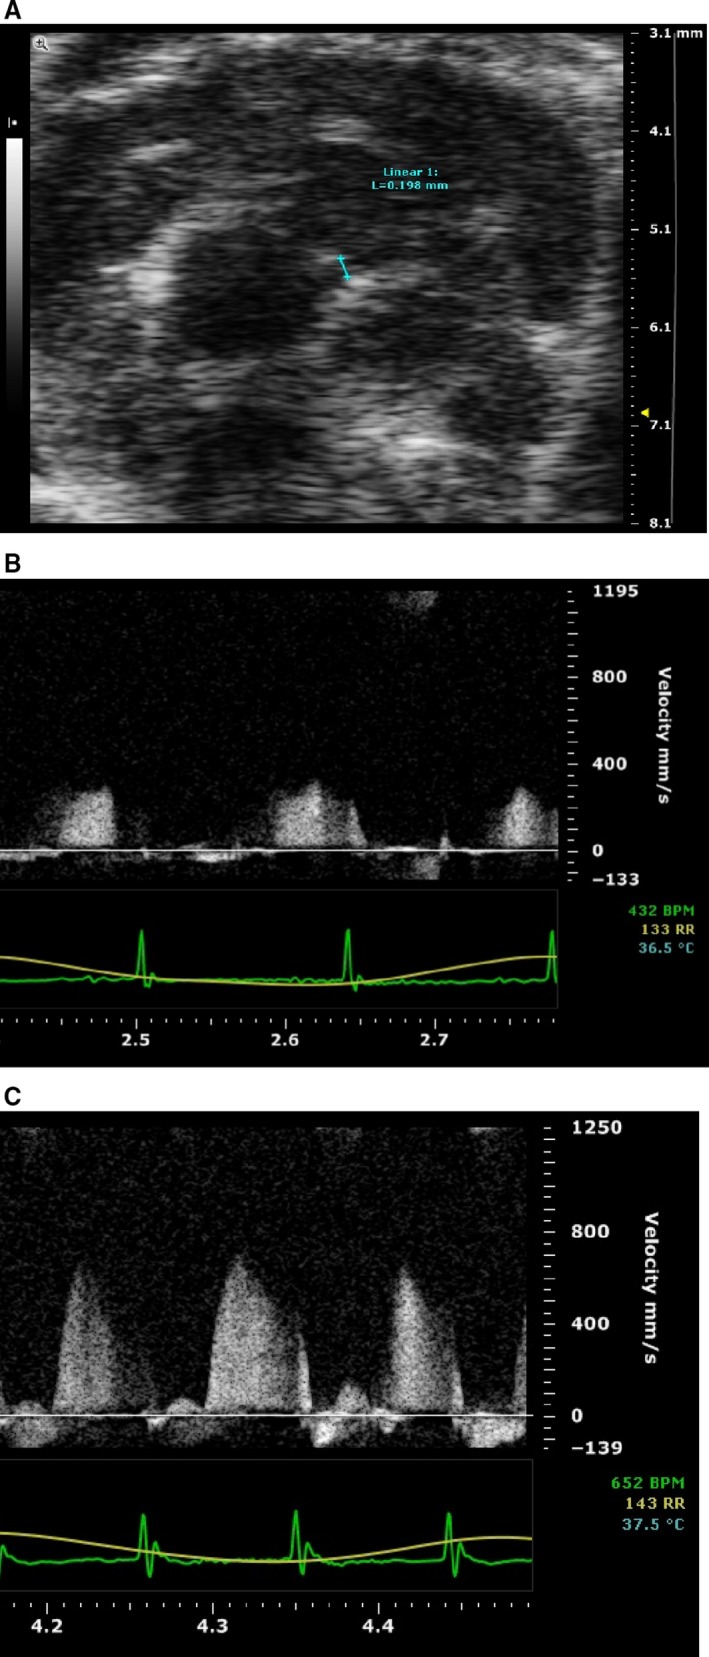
Sample ultrasound images for (A) size of coronary artery; (B) baseline pulse wave Doppler; (C) adenosine‐induced increase in pulse wave Doppler.
